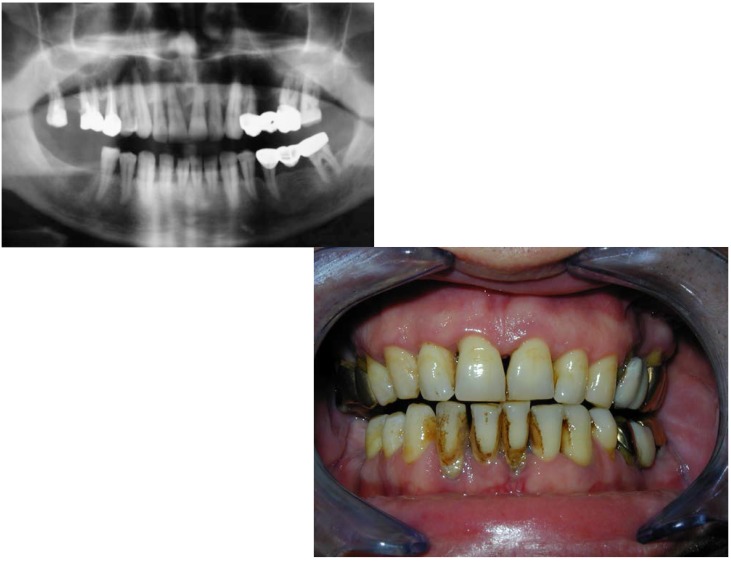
Preop x-ray and intraoral view of a 47 years old patient. He accused pain and mobility of tooth 1.1. Before the surgery a throughout cleaning is performed.
